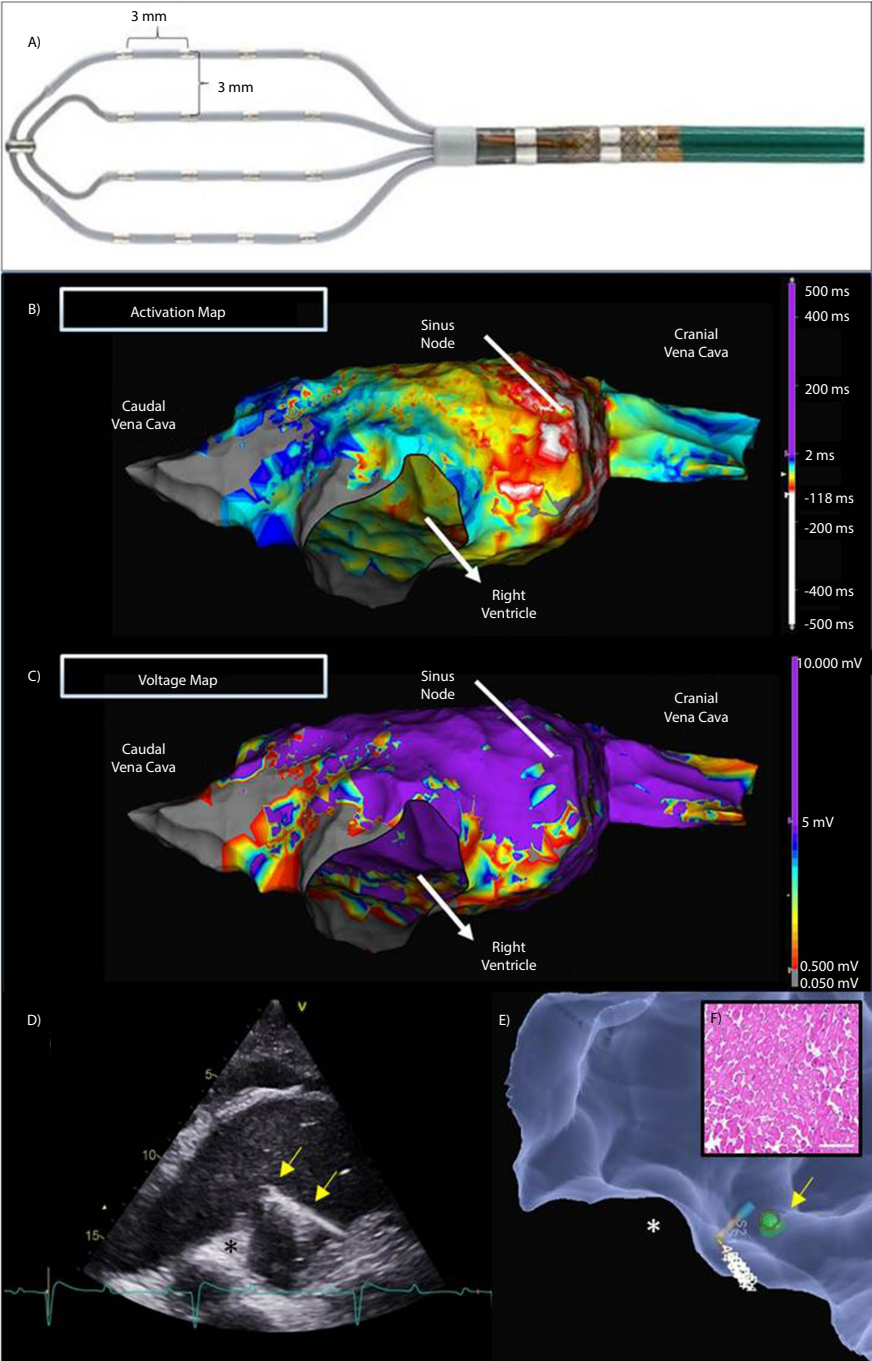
Advisor™ HD Grid Mapping Catheter (A, E). Representative activation (B) and voltage (C) map of the right atrium. Colour scale bar indicates activation time (ms) with the earliest activation in the sinus node (B) and voltage amplitudes (mV) of local electrograms (C). Biopsy forceps (yellow arrow) was used to collect tissue (F) from areas at interest. The biopsy forceps (yellow arrow) could be located both on echocardiography (D) and in the EnSite Velocity^™^ system (green mark, E). *Intervenous tubercle
